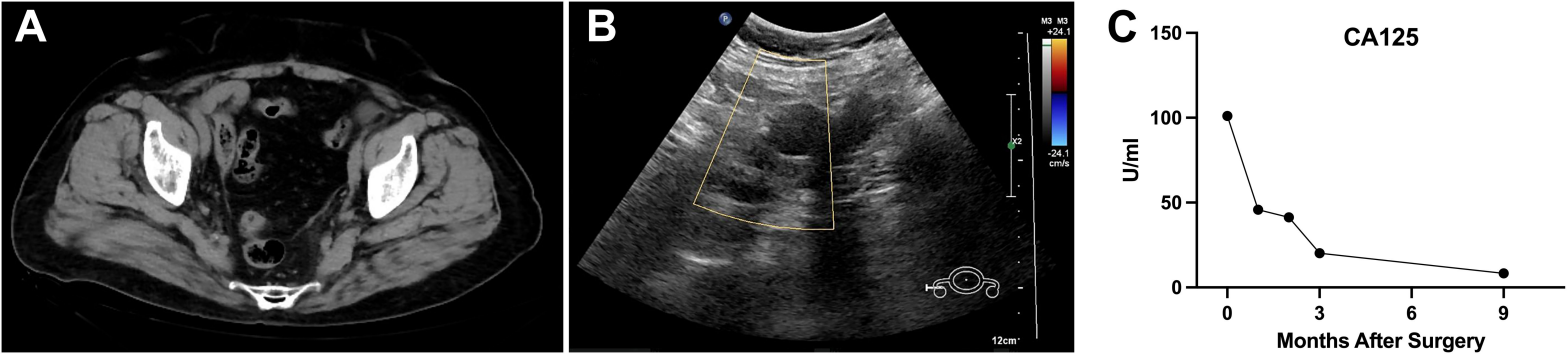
Postoperative follow-up imaging and tumor marker trends indicating no recurrence or metastasis. (A, B) Postoperative follow-up pelvic CT and gynecological ultrasound performed 9 months after surgery revealed no signs of recurrence or metastasis in the pelvic region. (C) Serial measurements of the tumor marker CA125 demonstrated a continuous downward trend post-surgery. CA125, cancer antigen 125.
